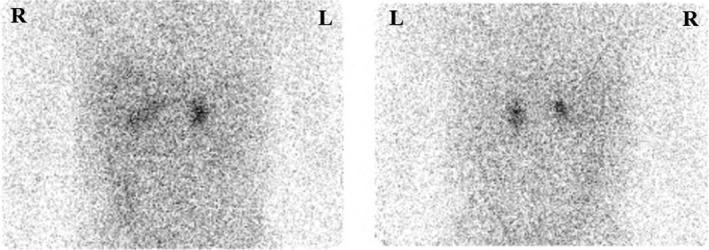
^131^I‐adosterol scintigraphy under dexamethasone suppression showed a bilateral adrenal uptake
